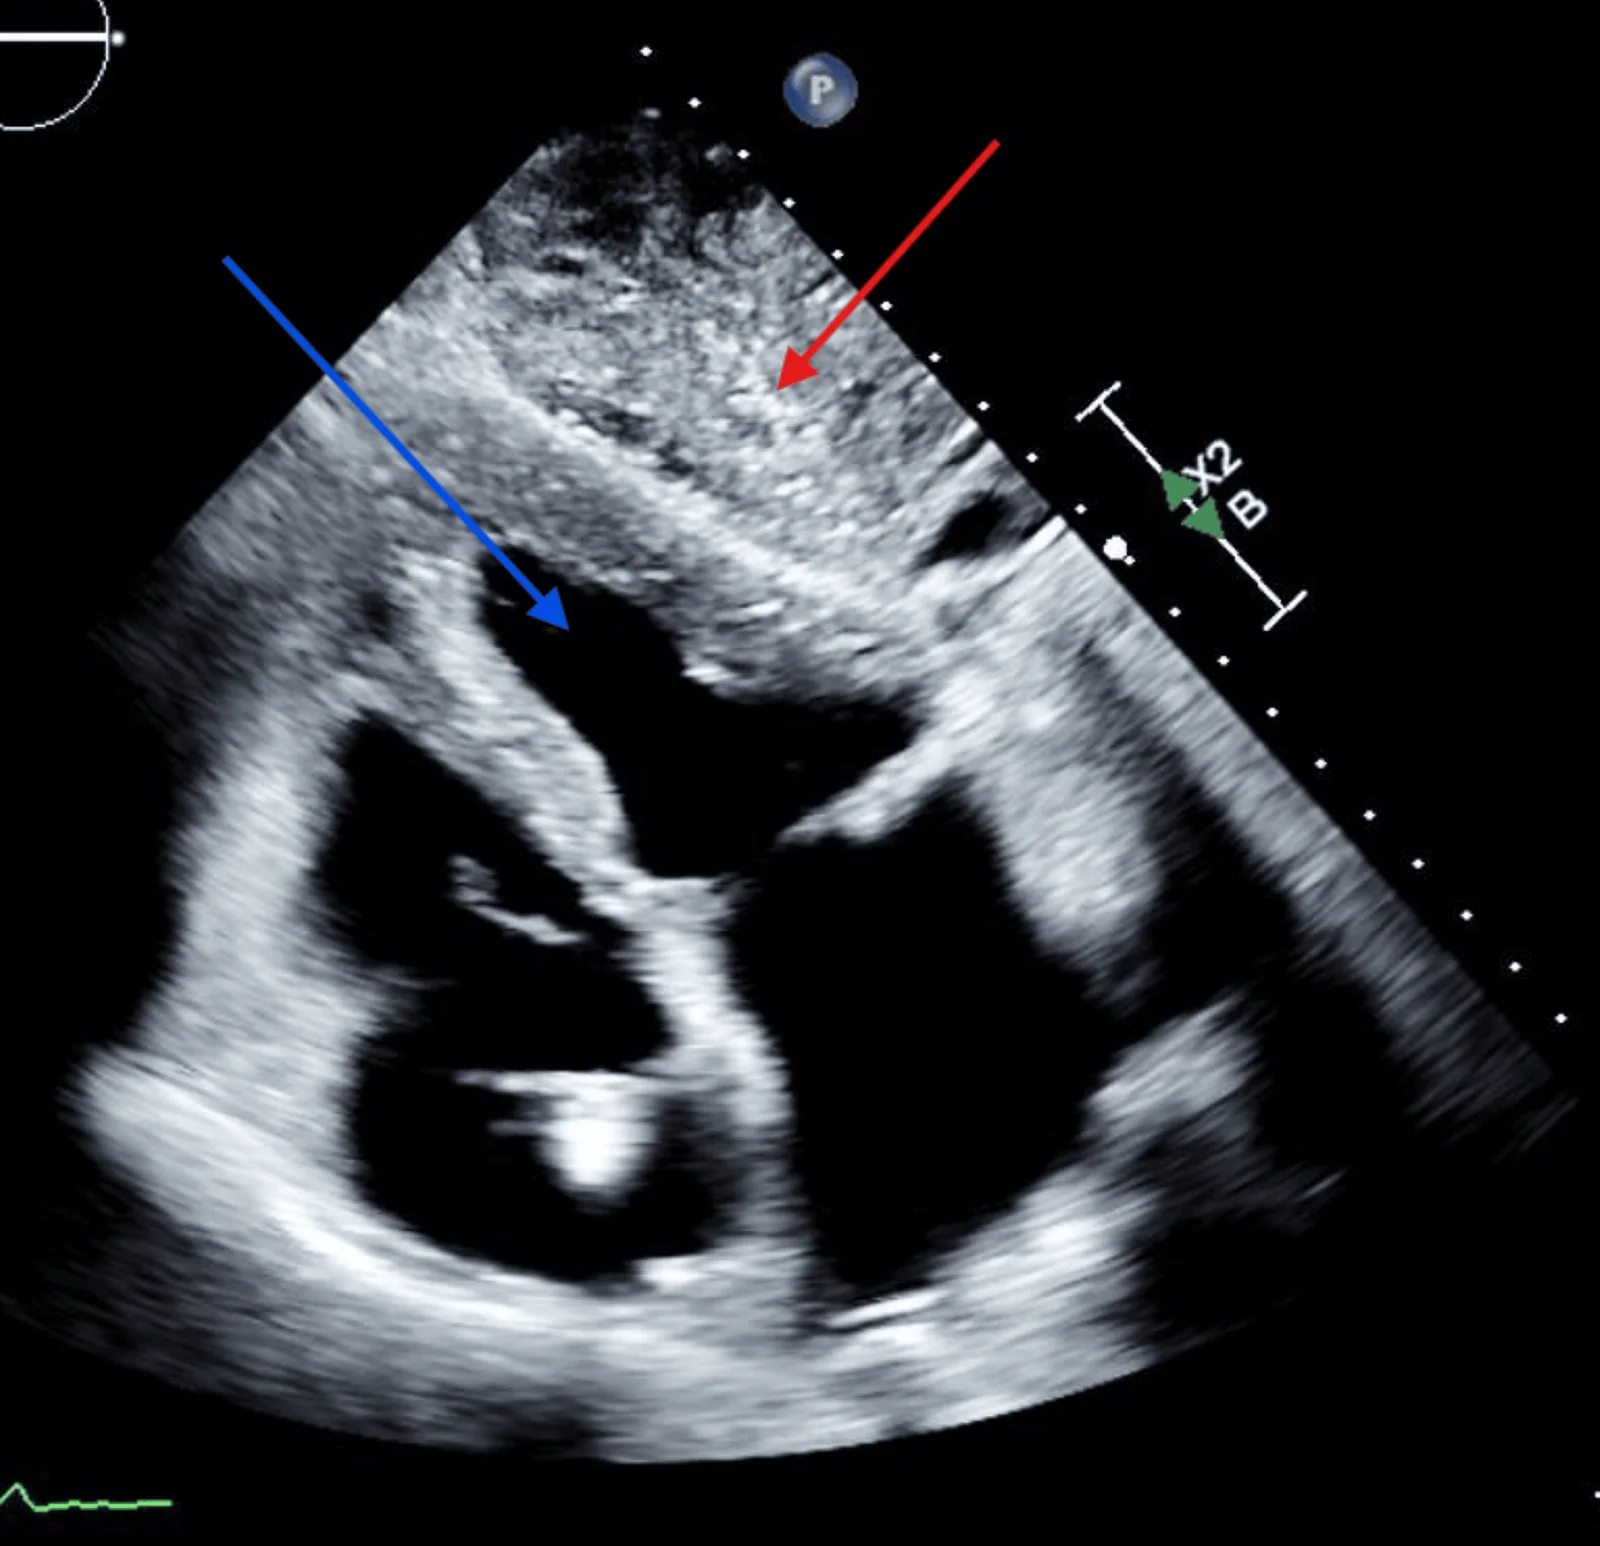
Echocardiogram in systole showing left ventricle (blue arrow) and pleural haematoma (red arrow).
